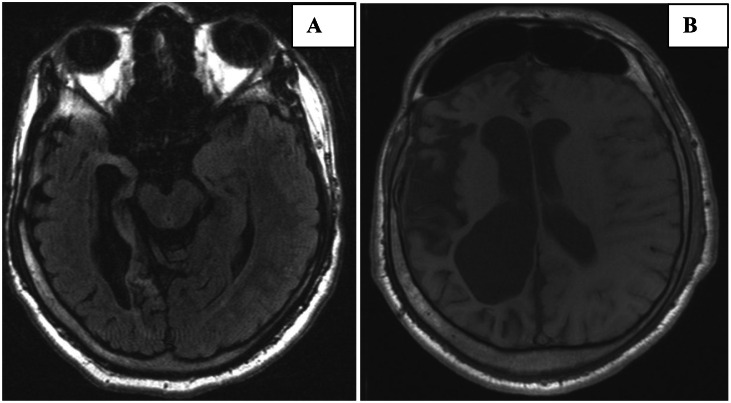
(A) Axial FLAIR image demonstrates asymmetric atrophy of the right cerebral peduncle, consistent with Wallerian degeneration. (B) Axial T1-weighted MRI shows prominence of the right frontal sinus with asymmetric thickening of the right calvarium.
